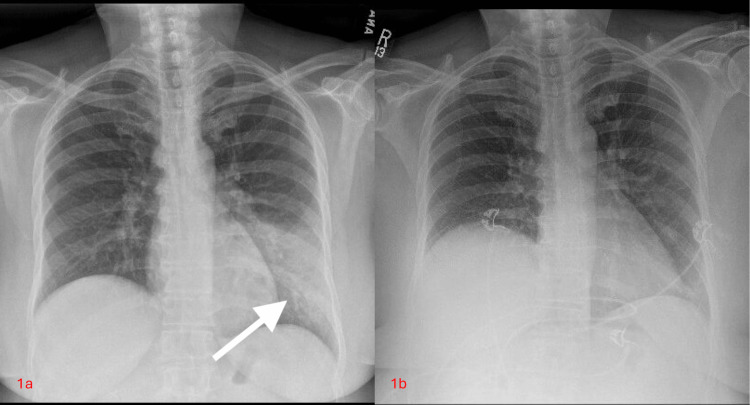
(a) Admission chest radiograph demonstrating left lower lobe consolidation. (b) Prior baseline chest radiograph for comparison.
